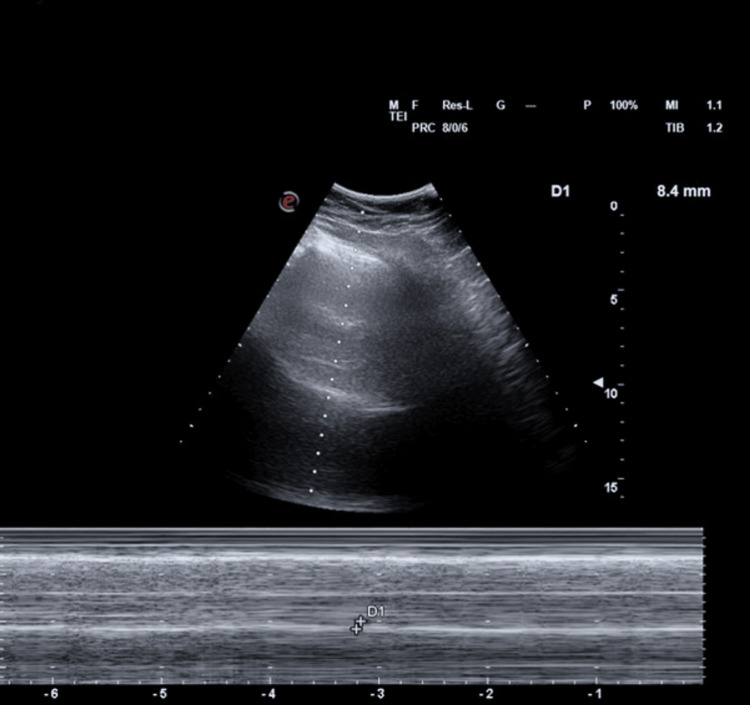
Pleural ultrasound, showing reduced excursion of the right hemidiaphragm.
